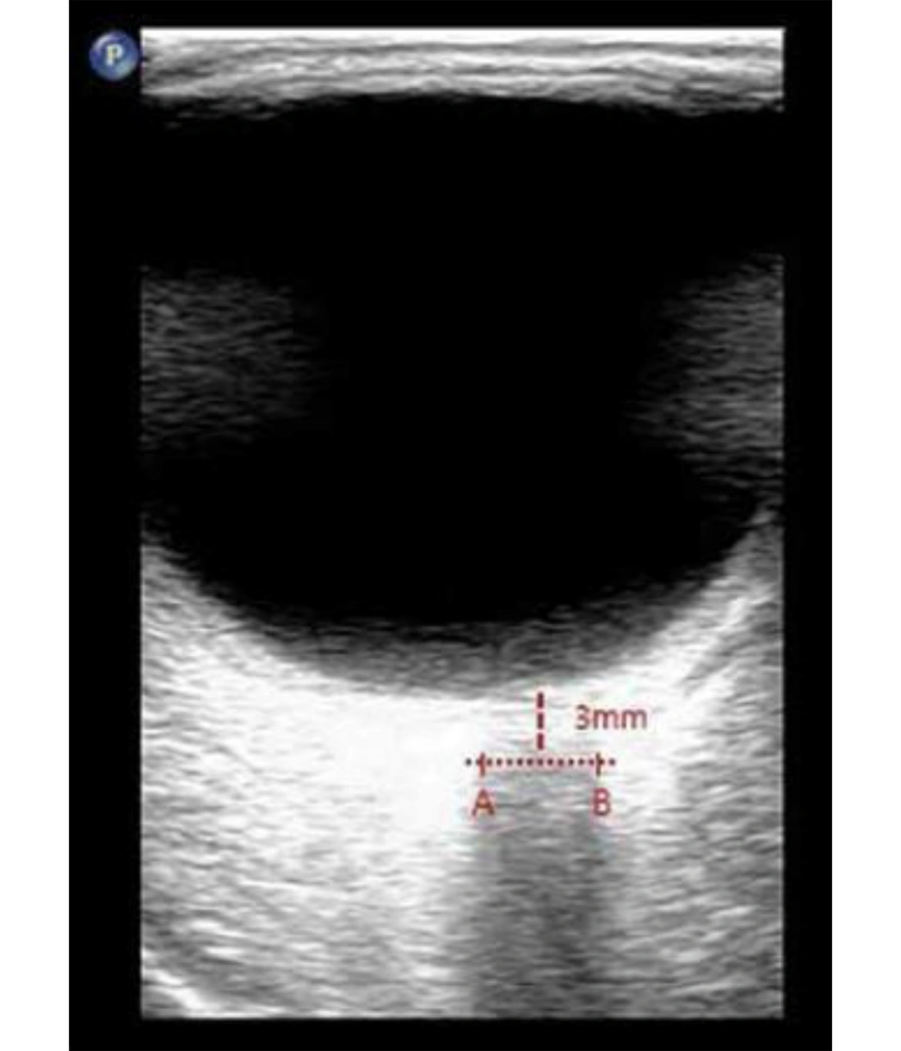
Optic nerve sheath diameter (ONSD) measurement by ultrasonography. The ONSD was measured by 3 mm posterior to the optic nerve head (A and B) in the axial image of the orbit in the plane of the optic nerve.
